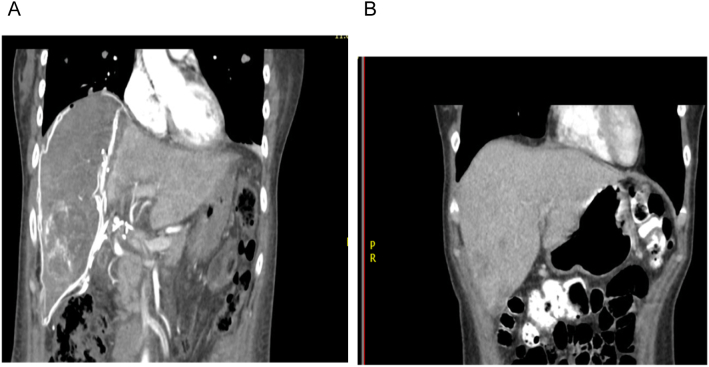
A: 7 day postoperative abdominal CT. Left lobe of the liver with 51% of hypertrophy (coronal view). B: Pre-operative abdominal CT. Comparison of the left lobe (coronal view).
